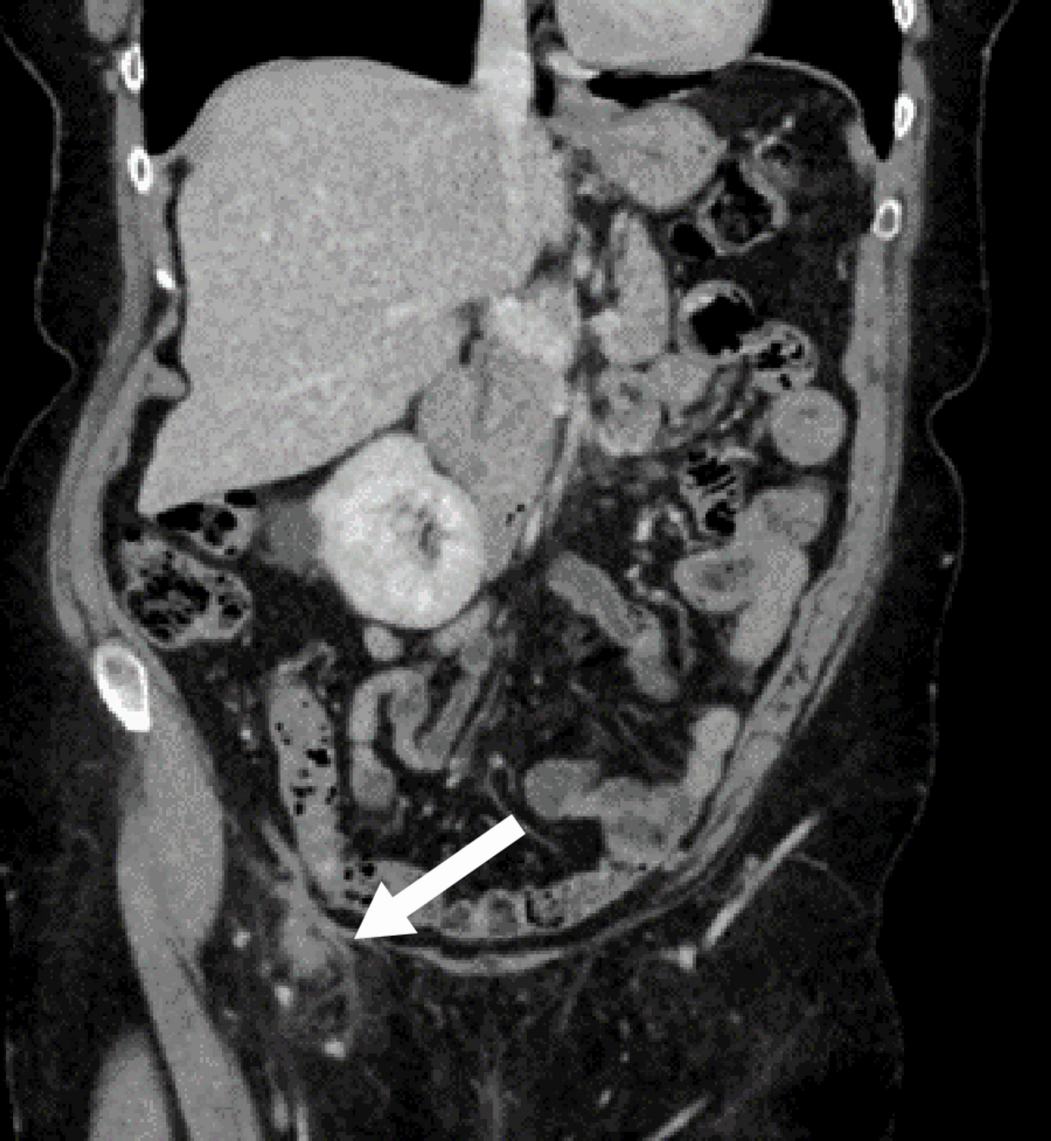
Coronal view of the CT scan of the abdomen and pelvis showing a right groin swelling (white arrow) that contains a pocket of fluid with a sac measuring 41 × 36 mm with no involvement of the bowel loops CT: computed tomography
